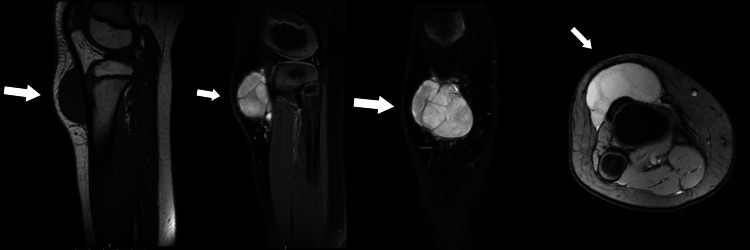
MRI images show an oval lesion on subcutaneous area in front of the tibial tubercle with increased signal intensity on T2-weighted images and low signal intensity on T1-weighted images. There are thin internal septa with smooth external contour and no bone involvement. There is absence of surrounding edema (arrows).
